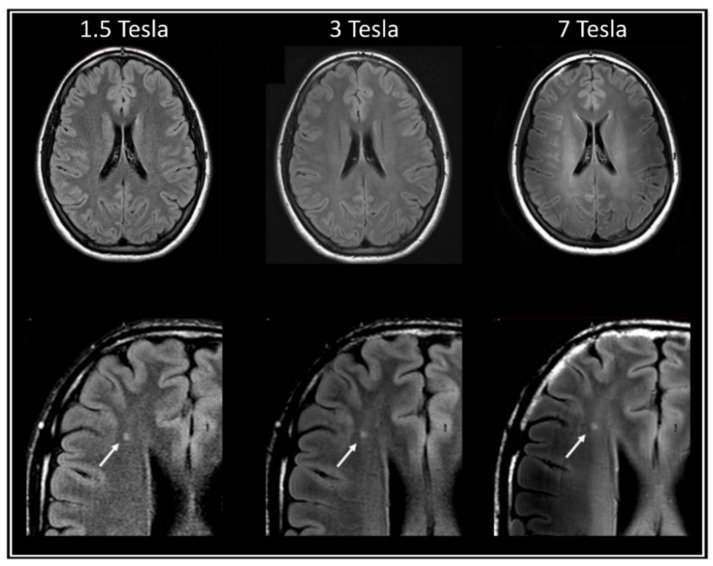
Impact of magnetic field strength and acquisition contrast on MRI images. Comparison of the effects of a 1.5, 3 and 7 Tesla magnetic field strength on image resolution for a T_2_-FLAIR-weighted image. The arrow indicates a white matter lesion (images from Zwanenburg, J.J.M. et al. [177], obtained with permission).
